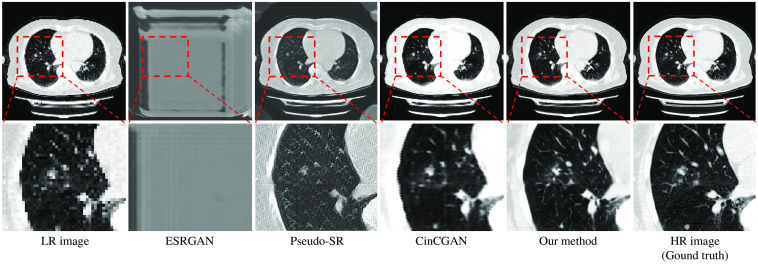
Experimental result on COVID-19 Lung CT Lesion Segmentation Challenge—2020 dataset.^52^ We compared our method with ESRGAN,^51^ pseudo-SR,^49^ and CinCGAN.^32^ It is noteworthy that because our method is trained with unpaired LR-HR images pairs, we also train ESRGAN with unpaired LR-HR images. ESRGAN output unreasonable results. Pseudo-SR and CinCGAN output blurry and noisy results. On the other hand, our method output convincing results. Upper: whole images from the axial axis. Lower: zoom-in on regions in the red boxes for better comparison.
